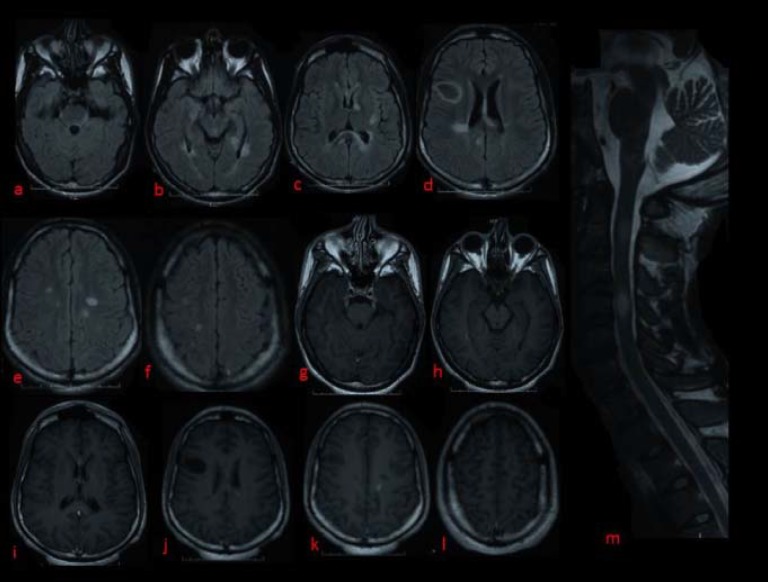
Magnetic resonance imaging (MRI) findings prior to therapy. Axial sections of cerebral MRI FLAIR show hyperintense periventricular and subcortical lesions and a tumor-like frontal lesion with peripheral open ring gadolinium enhancement (g, h, I, j, k, l), axial sections of cerebral MRI in post-contrast T1 sequences show 3 actives lesions with contrast enhancement (a, b, c, d, e, and f), and longitudinal section of the spinal cord in T2 sequence show 2 cervical hyperintense lesions (m).
